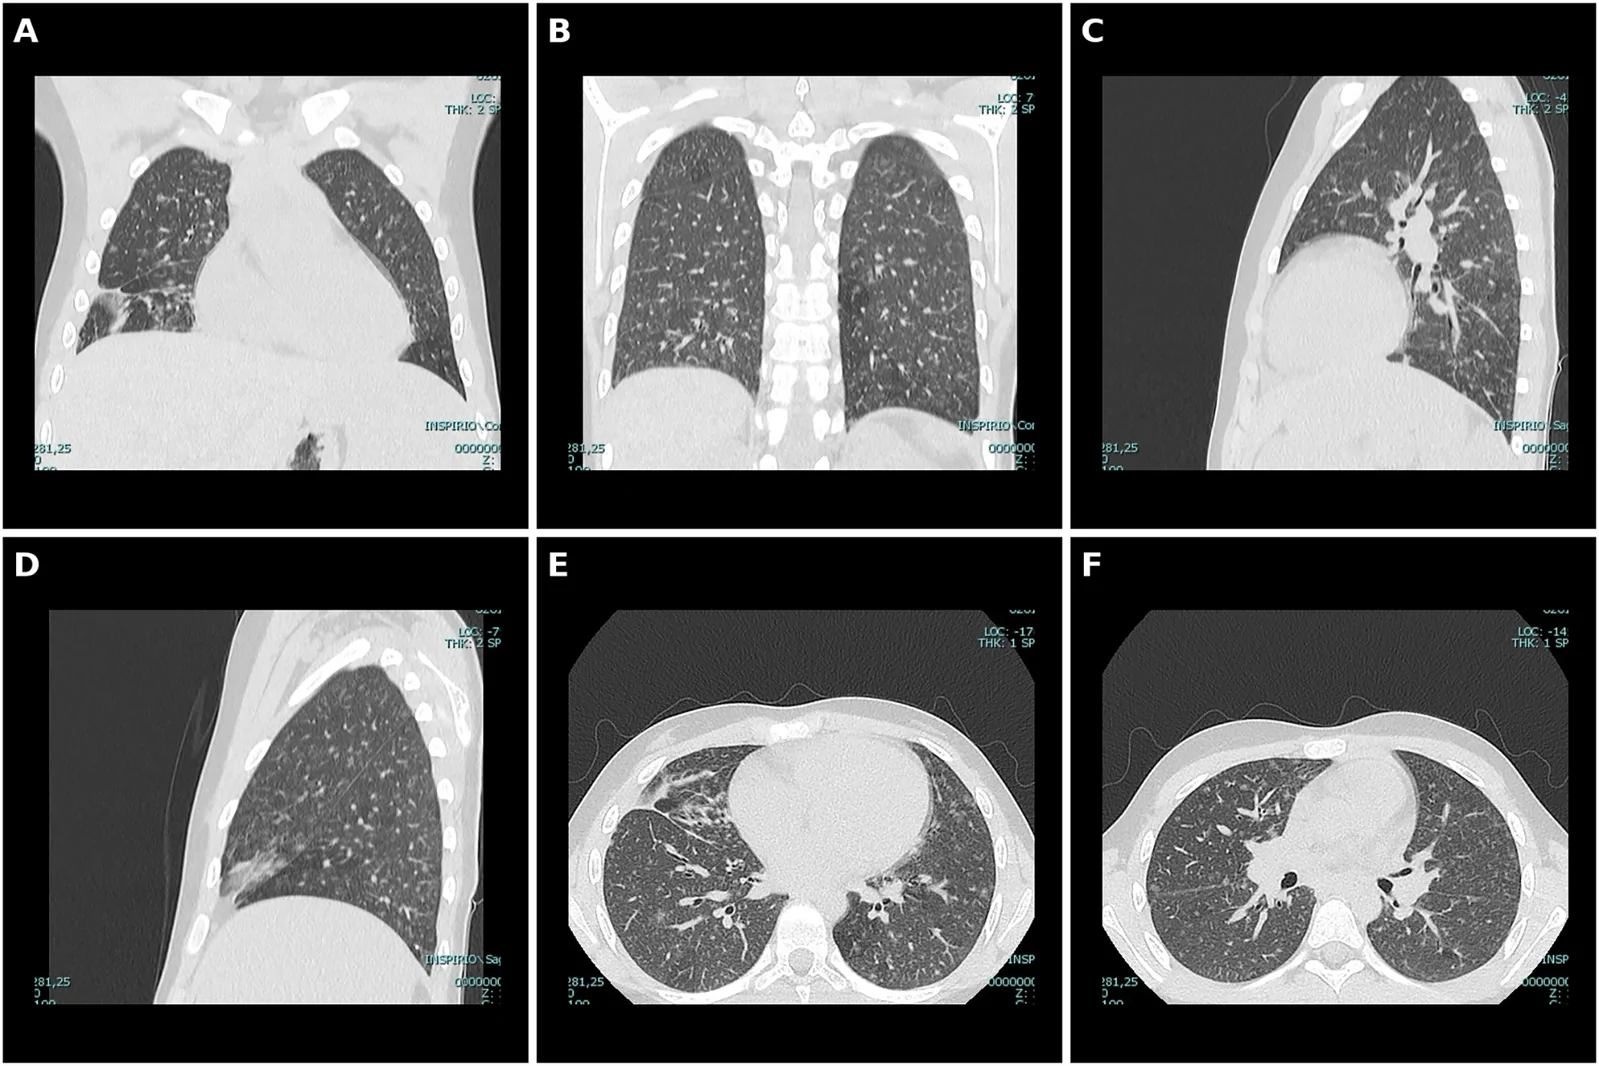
Chest computed tomography findings. (A–B) Coronal CT scan showing bilateral diffuse interstitial involvement. (C–D) Sagittal CT scan highlighting peribronchovascular thickening and patchy ground-glass opacities. (E–F) Axial CT scan showing subpleural consolidations and ground-glass opacities.
